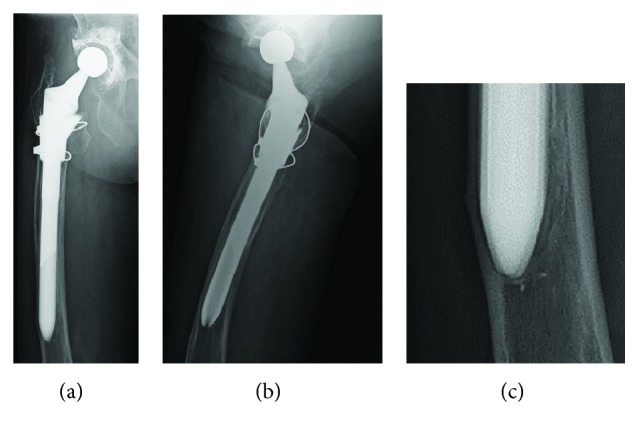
(a) Anteroposterior radiograph, (b) mediolateral radiograph, and (c) magnified anteroposterior radiographs of the fracture line. Five months before hospitalization, the cortical bone was thinning slightly in proximity to the tip of the stable femoral stem and the transverse lucency was admitted on the outside.
